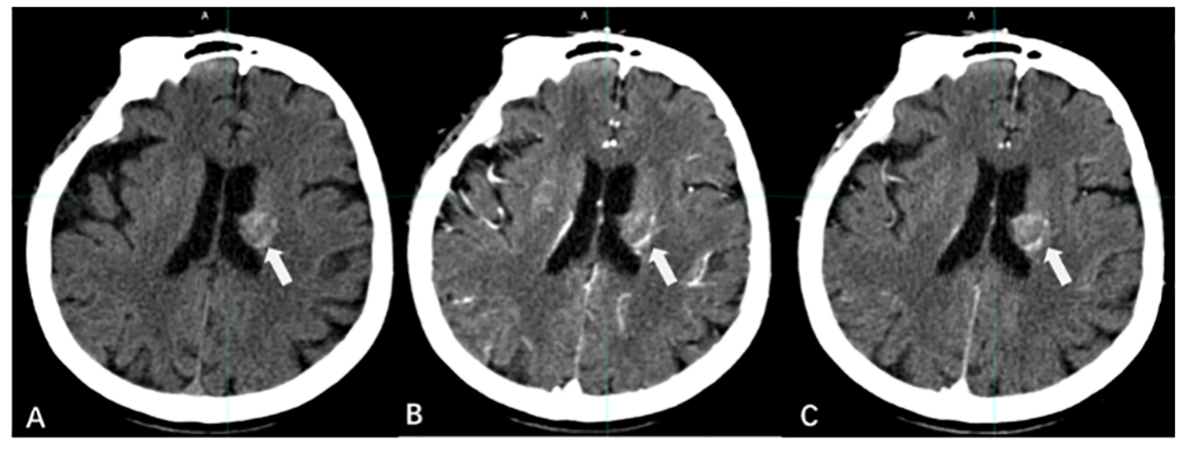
CT imaging manifestations of BMs. [(A) CT scan without contrast; (B) CT contrast scan in arterial phase; (C) CT contrast scan in vein phase].
